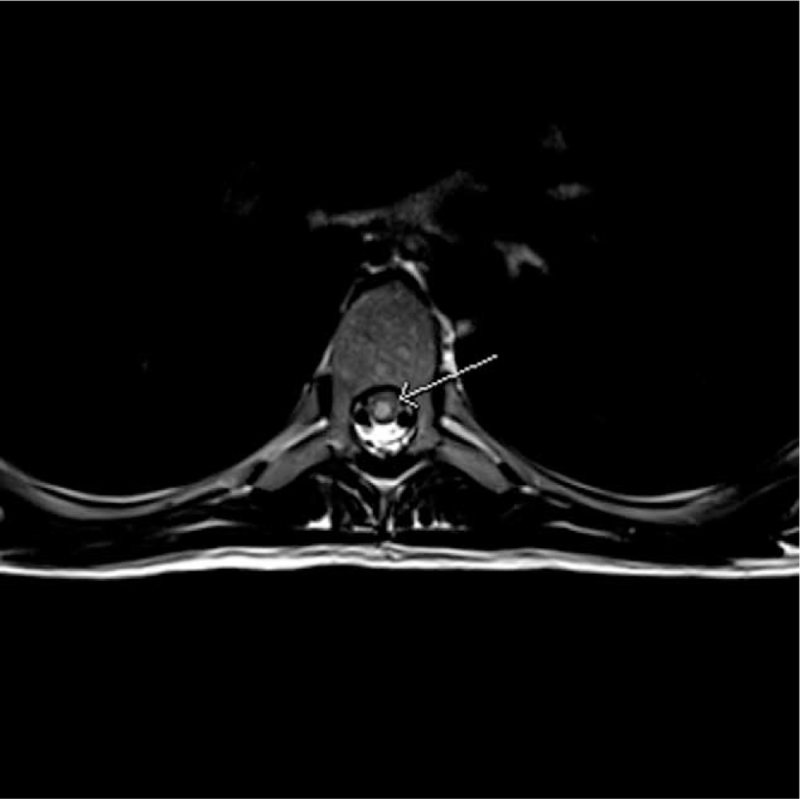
Axial T2 sequence image of MRI spine demonstrated increase signal in central cord at T6 level. MRI = magnetic resonance imaging.
